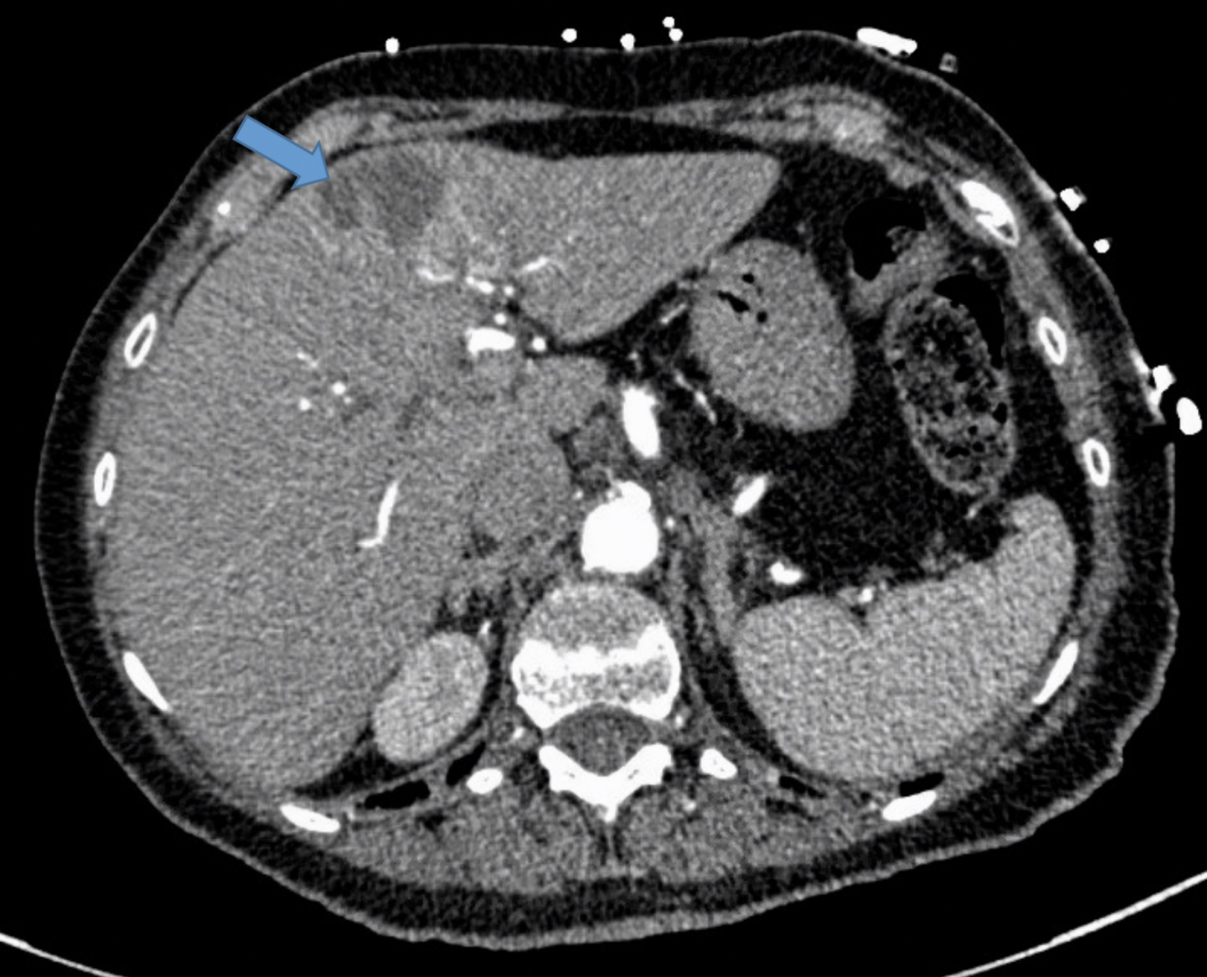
Computed tomography scan of the abdomen revealing 2 x 3 cm hypodensity in the left lobe of the liver.
